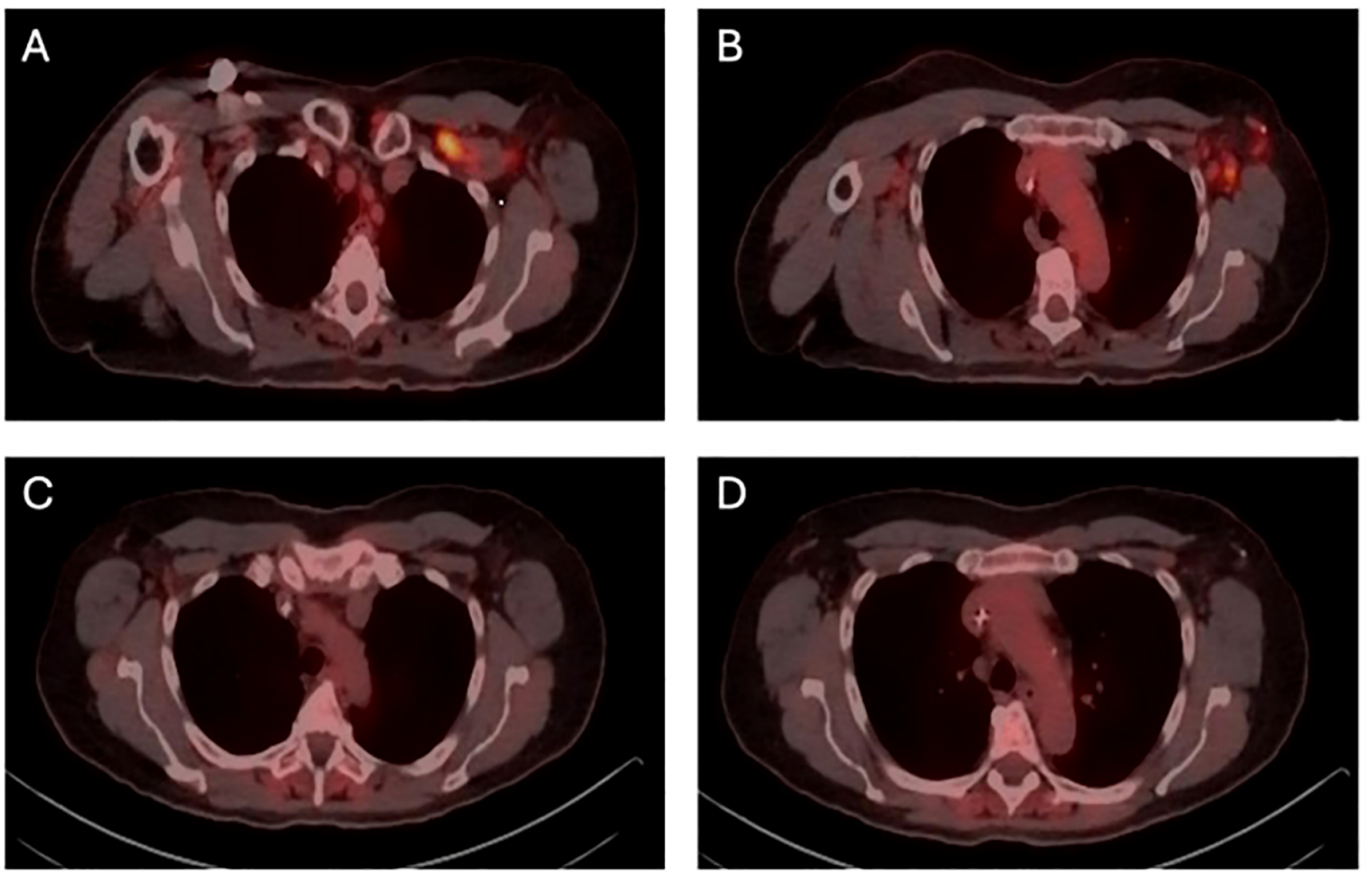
FDG-PET (fluorodeoxyglucose-positron emission tomography) demonstrating metabolic response to systemic therapy. (A) initial FDG-PET prior to neoadjuvant chemotherapy, showing uptake in superior axillary (levels I, II, and III) lymph nodes (B) initial FDG-PET prior to neoadjuvant chemotherapy, showing uptake in inferior axillary (level I and II) lymph nodes. (C) re-staging FDG-PET following neoadjuvant chemotherapy, showing metabolic complete response in superior axillary lymph nodes. (D) re-staging FDG-PET following neoadjuvant chemotherapy, showing metabolic complete response in inferior axillary (levels I and II) lymph nodes.
